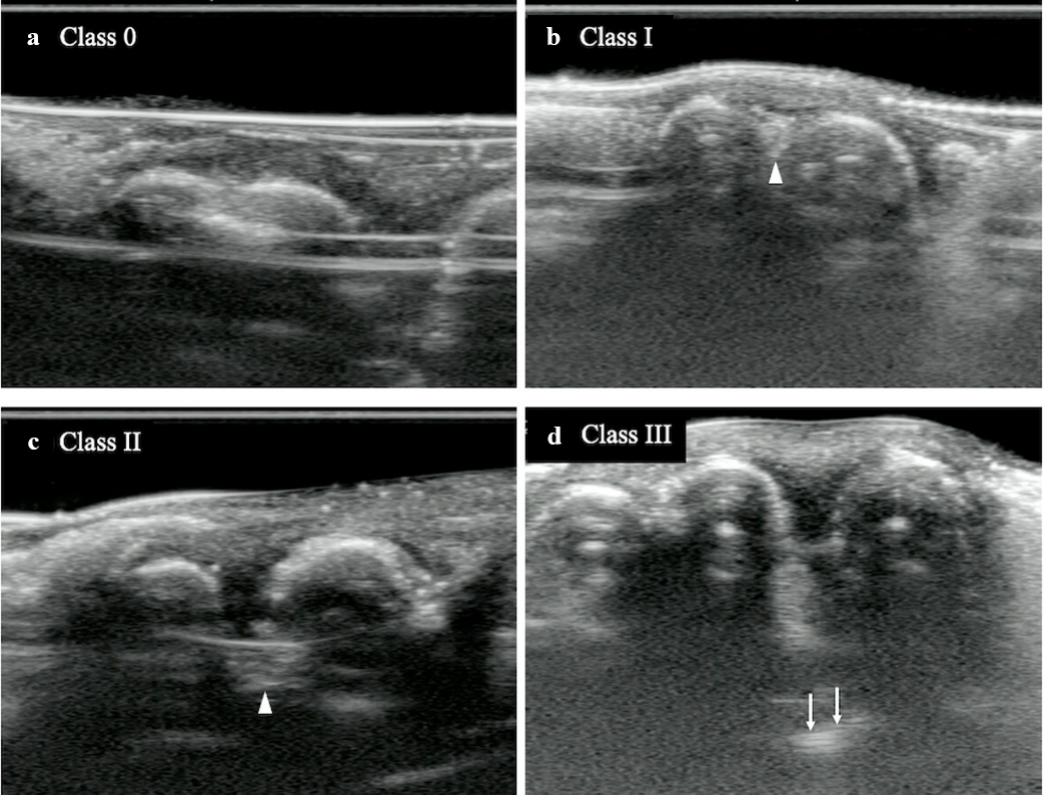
Classification of furcation involvement in B-mode intraoral USG. (A) Axial (Cross-sectional) image of a case without furcation involvement (Class 0). Interradicular part is not indicated. (B) Axial (Cross-sectional) image of a case classified in Class I. A V-shaped bone defect is observed between the roots. A white arrowhead indicates the end of bone defect. (C) Axial (Cross-sectional) image of a case classified in Class II. Considerably deep bone defect is demonstrated. A white arrowhead indicates the end of bone defect. (D) Axial (Cross-sectional) image of a case classified in Class III. Hyperechoic line from tongue mucosa (white arrows) is suspected at the end of interradicular bone defect. USG, ultrasonography.
